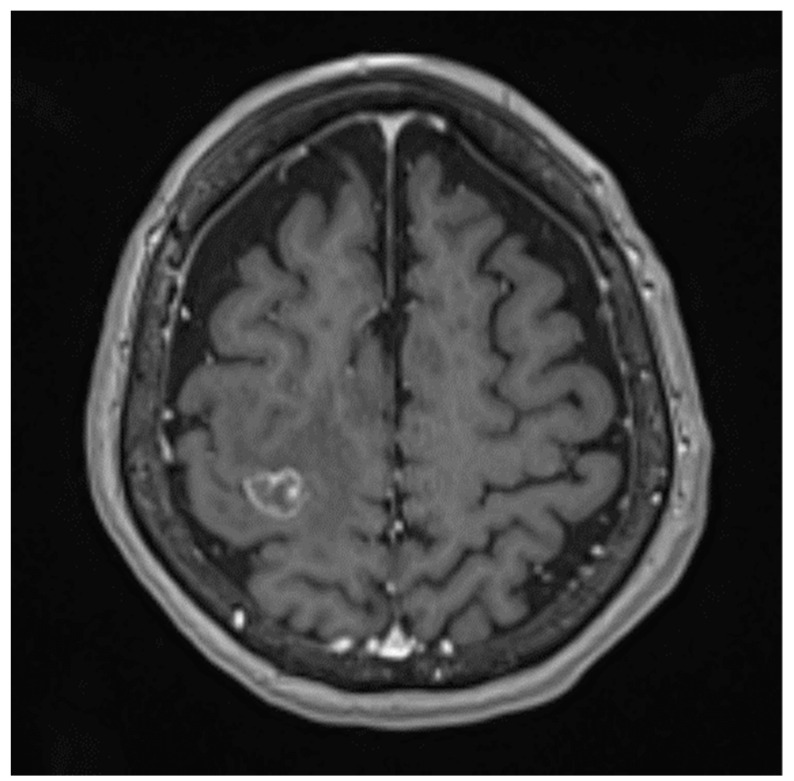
Solitary BrM on T1-weighted MRI with gadolinium.
